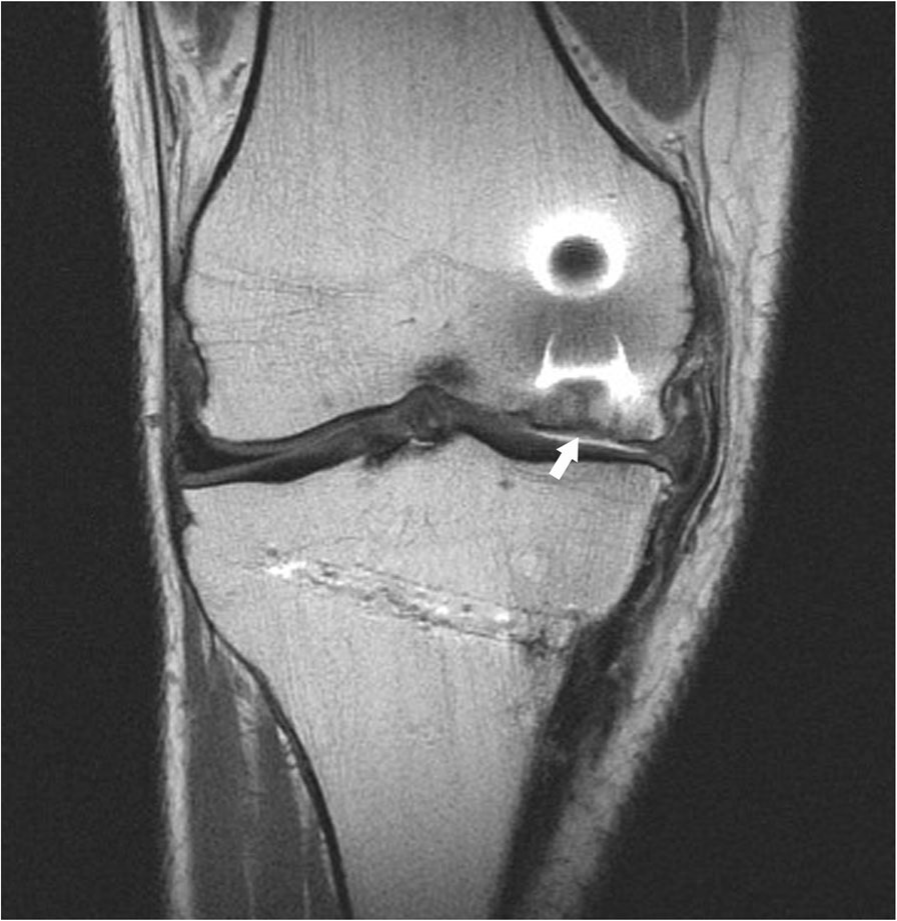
Magnetic resonance images obtained at follow-up. Images obtained in 2014 show the allograft integration into the medial condyle and the cartilage line under the graft (*white arrow*) 3 years after the intervention
